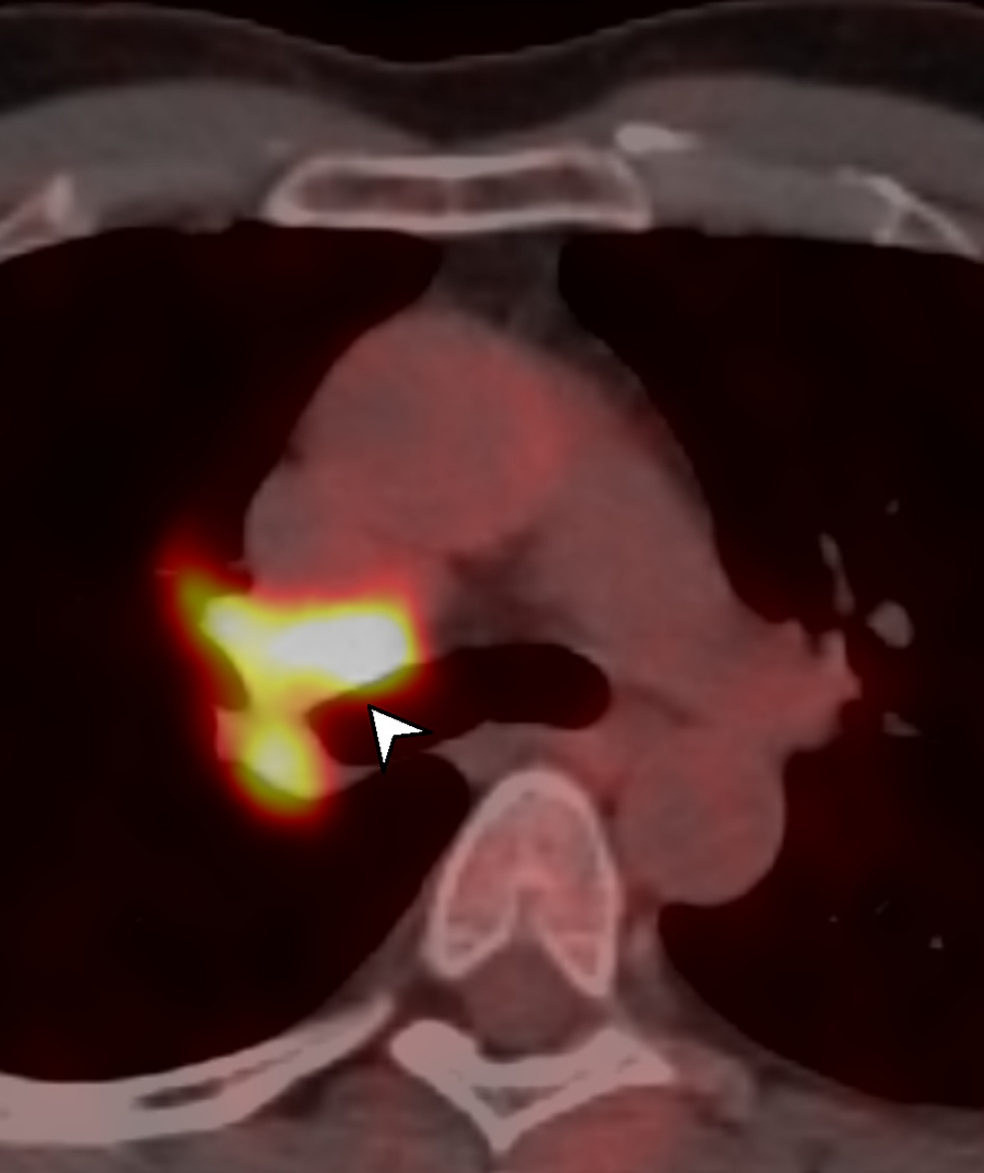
PET-CT showing a hypermetabolic lesion (white arrowhead) PET-CT: positron emission tomography-computed tomography
